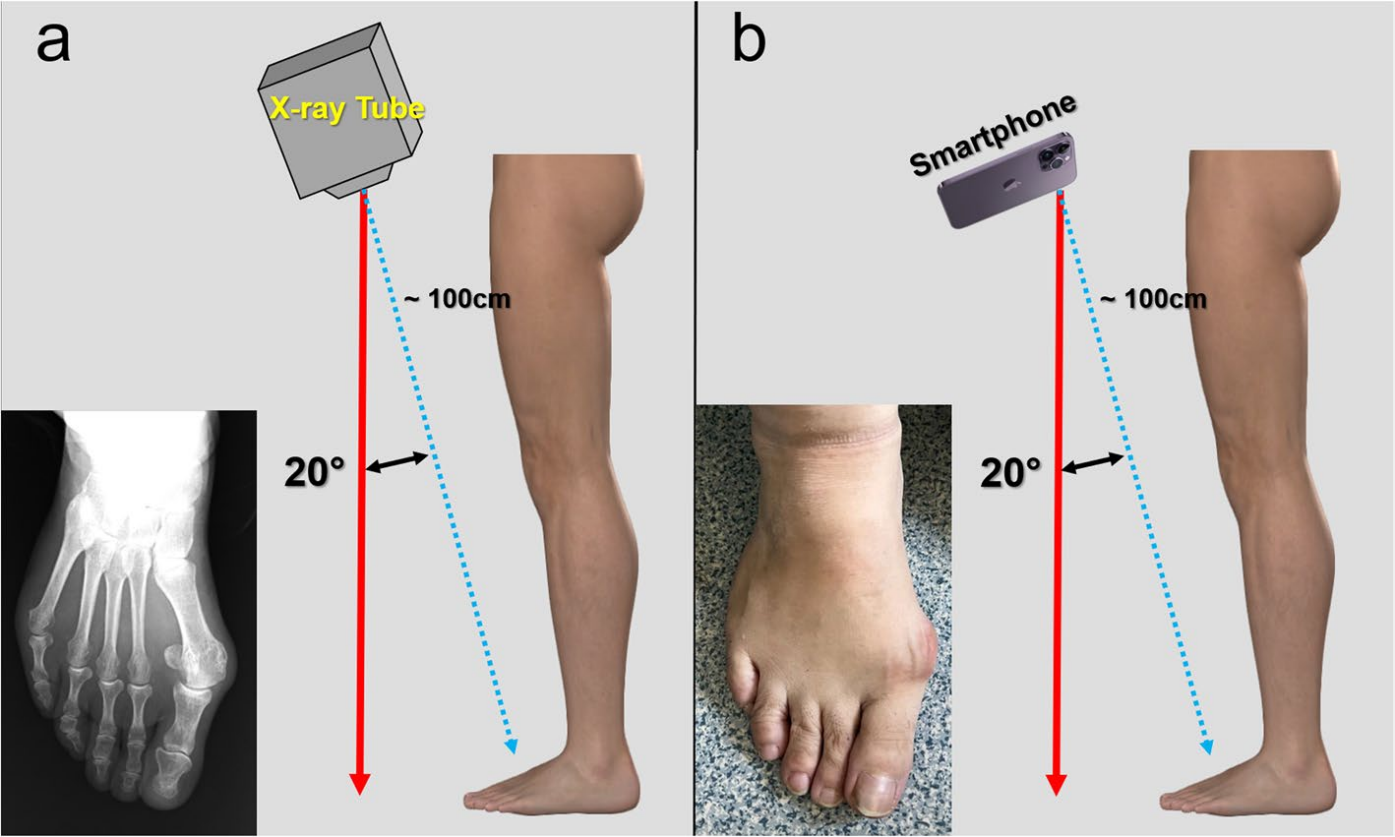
a Radiographic imaging technique. b Photographic imaging technique
